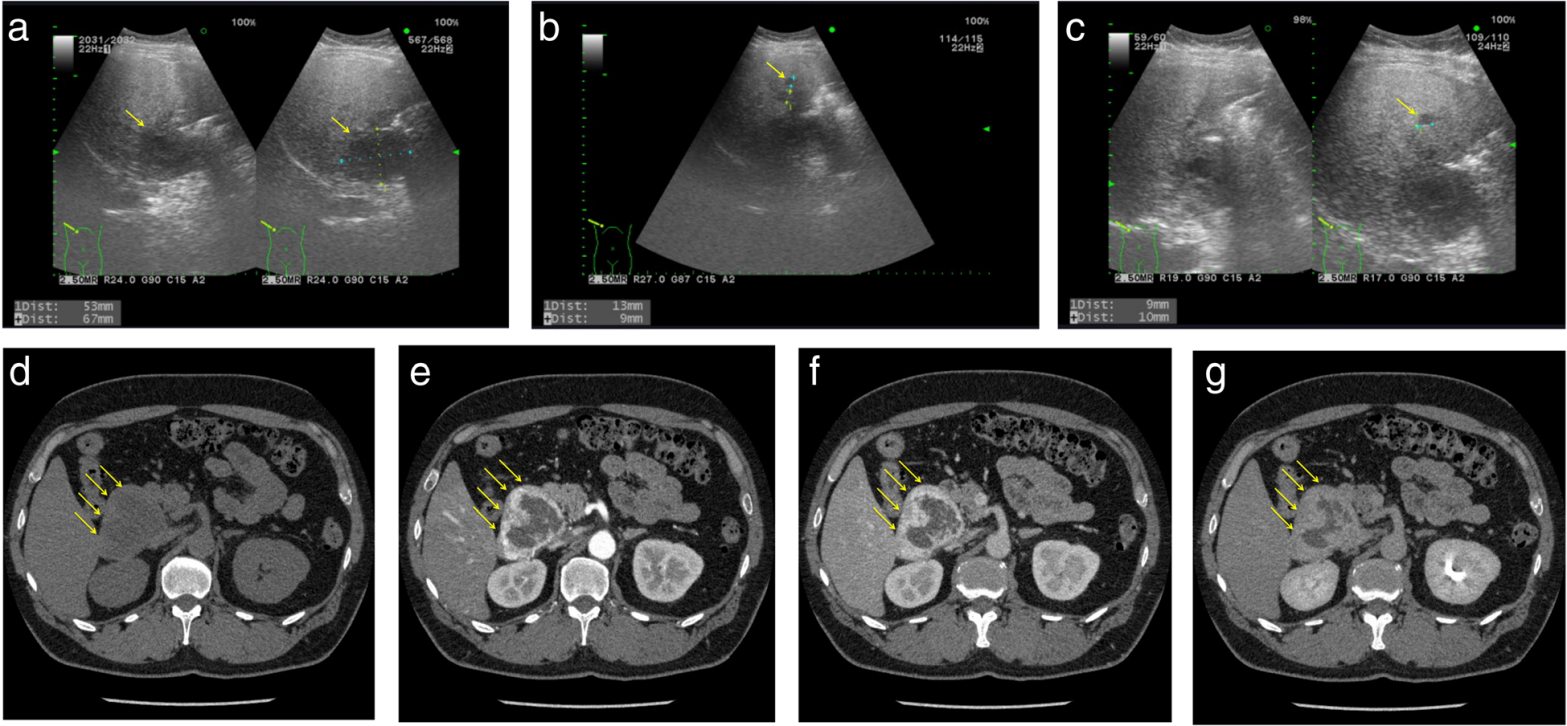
Abdominal ultrasonography (US) and contrast-enhanced abdominal computed tomography (CT). US images showing well-defined, hypoechoic masses in segments 1 (a), 2 (b), and 6 (c). Pre-contrast CT scan image showing a low-density 65-mm mass with an irregular margin in the caudate region (d). Contrast-enhanced CT scans images revealing peripheral early ring enhancement in the arterial phase and internal heterogeneous enhancement in the delayed phase (e–g)
